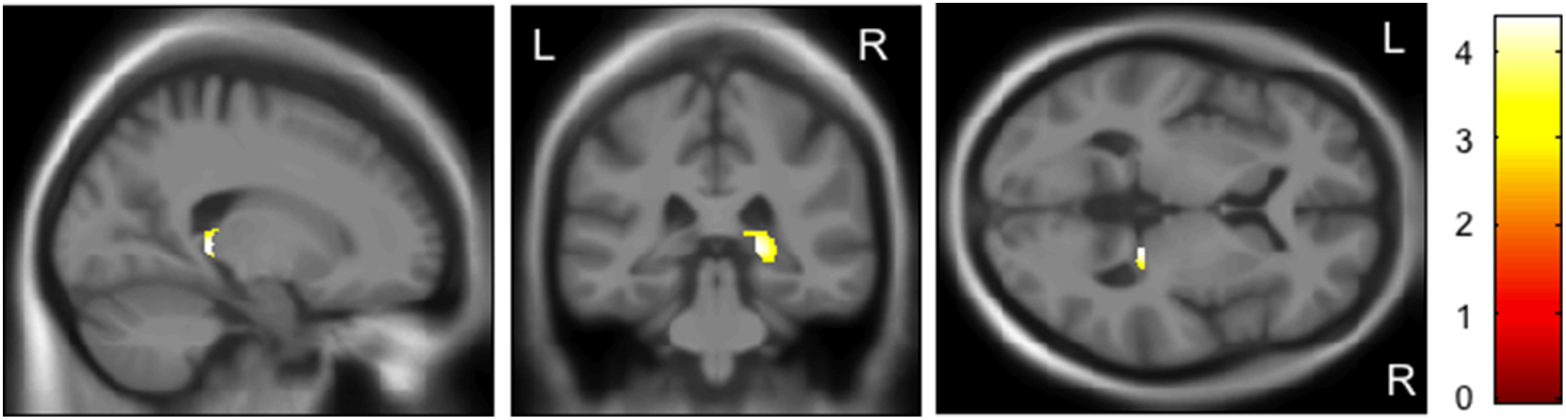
Impact of the *rs28365859* genotype on gray matter volume of the right hippocampus in schizophrenia. Age, sex, illness duration, and medication dose were used as covariates. The protective C allele carriers had a significantly larger right hippocampus than the G allele homozygotes. Anatomical localizations are displayed on the normal template MR images in three directions. The color bar shows t values corresponding to the color in the figure.
